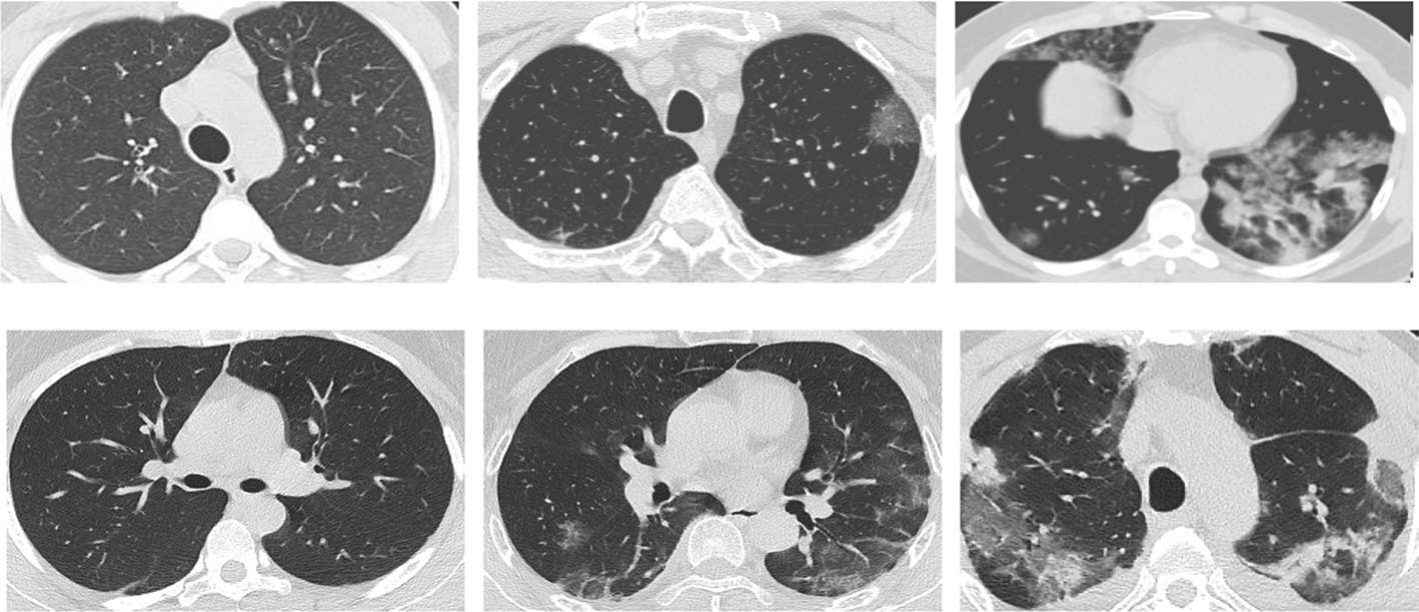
Chest CT sample images of COVID-19 negative  (column 1) and COVID-19 positive (columns 2&3) patients from the CoDS1 (row1) and the cropped CoDS2 (row 2) datasets
